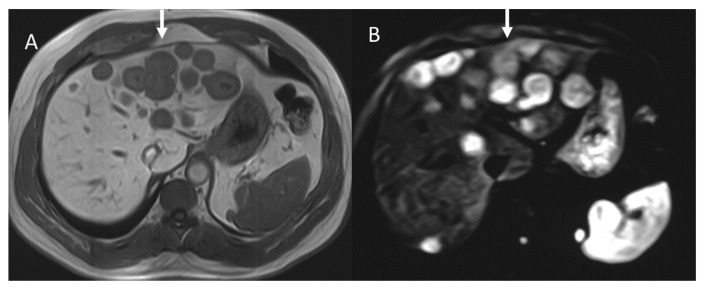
MRI evaluation of liver pNEN metastases. Morphological evaluation ((A): T1-W image) show hypointense lesions (arrow); in DWI ((B): b800 s/mm^2^), lesions show restricted signal.
